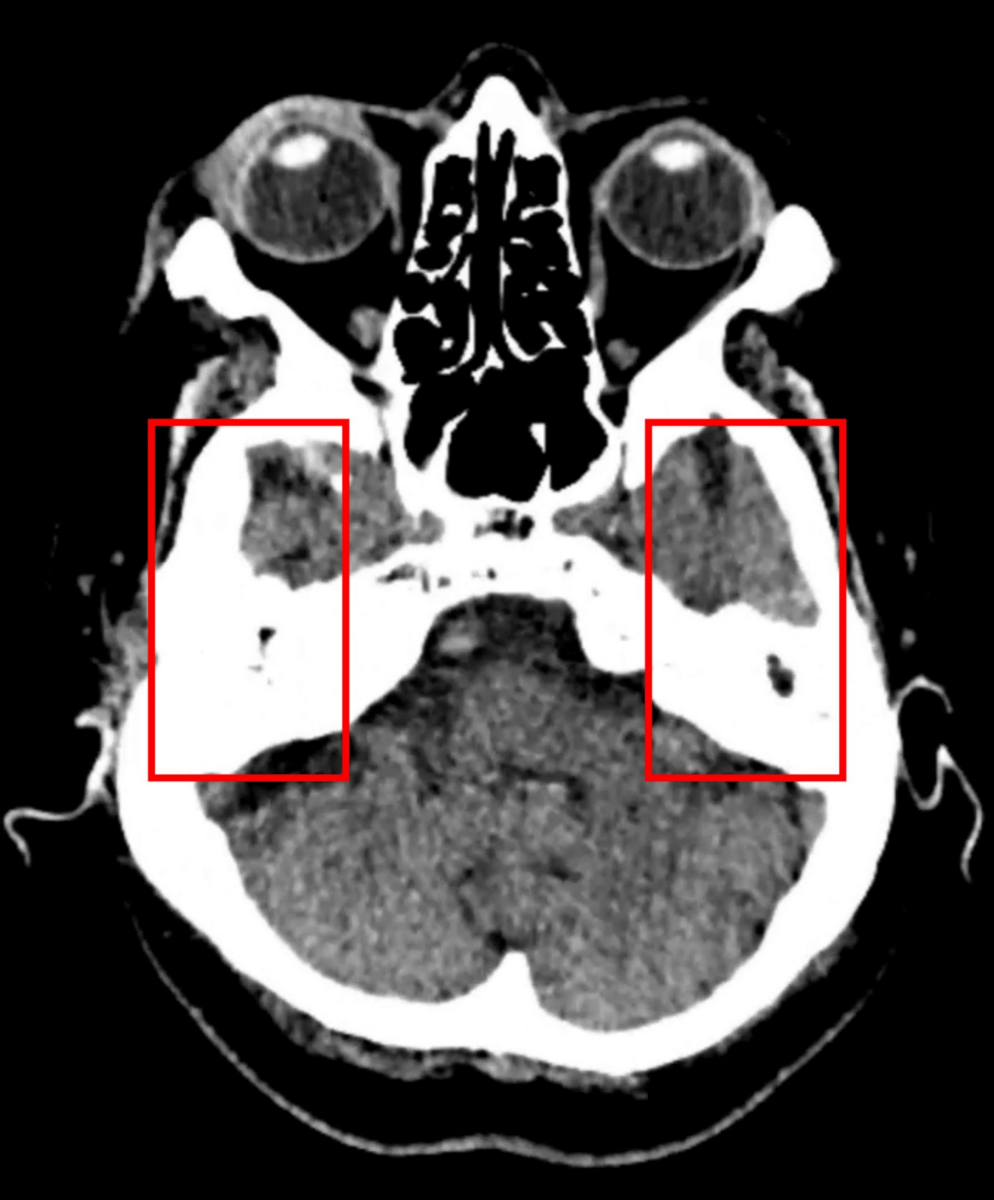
Axial CT showing bilateral mastoid opacification Axial CT demonstrating complete soft tissue opacification of both mastoid air cells without bony erosion, consistent with bilateral otomastoid inflammatory change.
